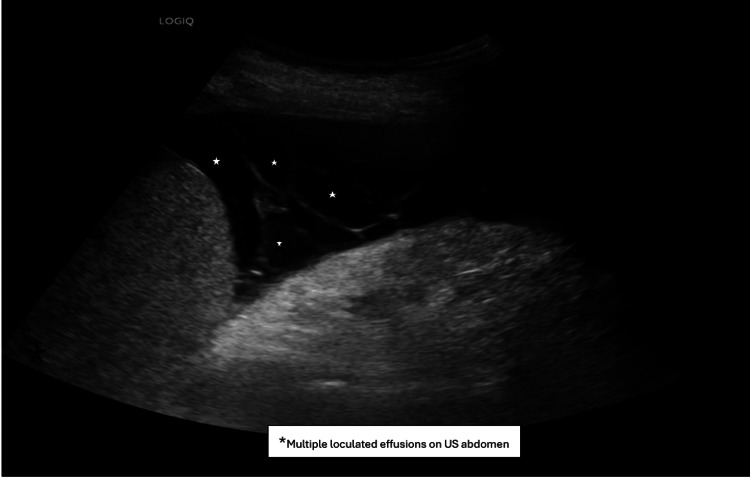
Ultrasound (US) abdomen showing loculated ascites
